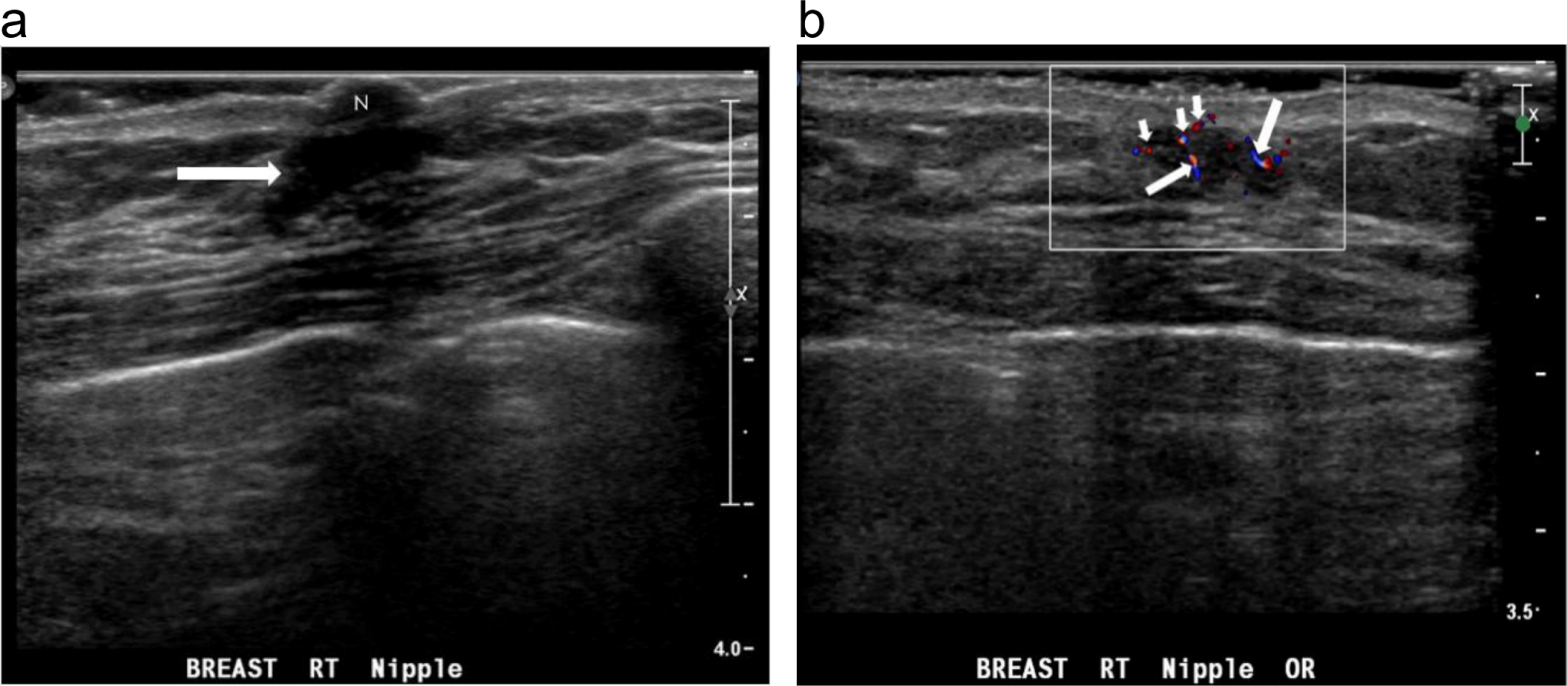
A nonpalpable nodule in the right breast of a 77-year-old man with bloody discharge from the right nipple. (a) Radial sonogram shows an irregular shape, a noncircumscribed margin, a mildly eccentric, homogeneously hypoechoic, subareolar nodule. The nodule has a parallel orientation and an abrupt interface. Neither an echogenic halo nor posterior features are present. (b) Transverse color Doppler ultrasound shows multiple color pixels (short arrows) and short pedicles of blood supply (long arrows) in the nodule, categorized as a rich color flow signal with predominantly internal vascularity. The postoperative pathological diagnosis is ductal carcinoma *in situ*.
